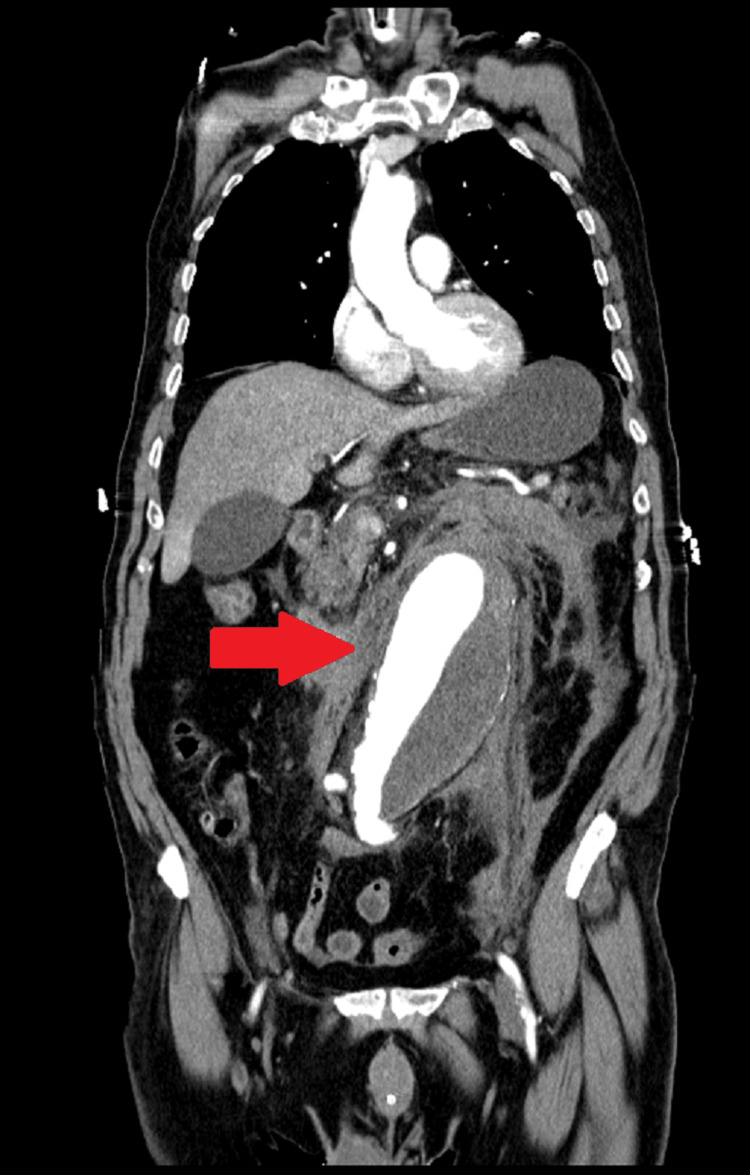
CT scan of the abdomen and pelvis with an aortic abdominal aneurysm and thrombus (arrow) CT: computed tomography
